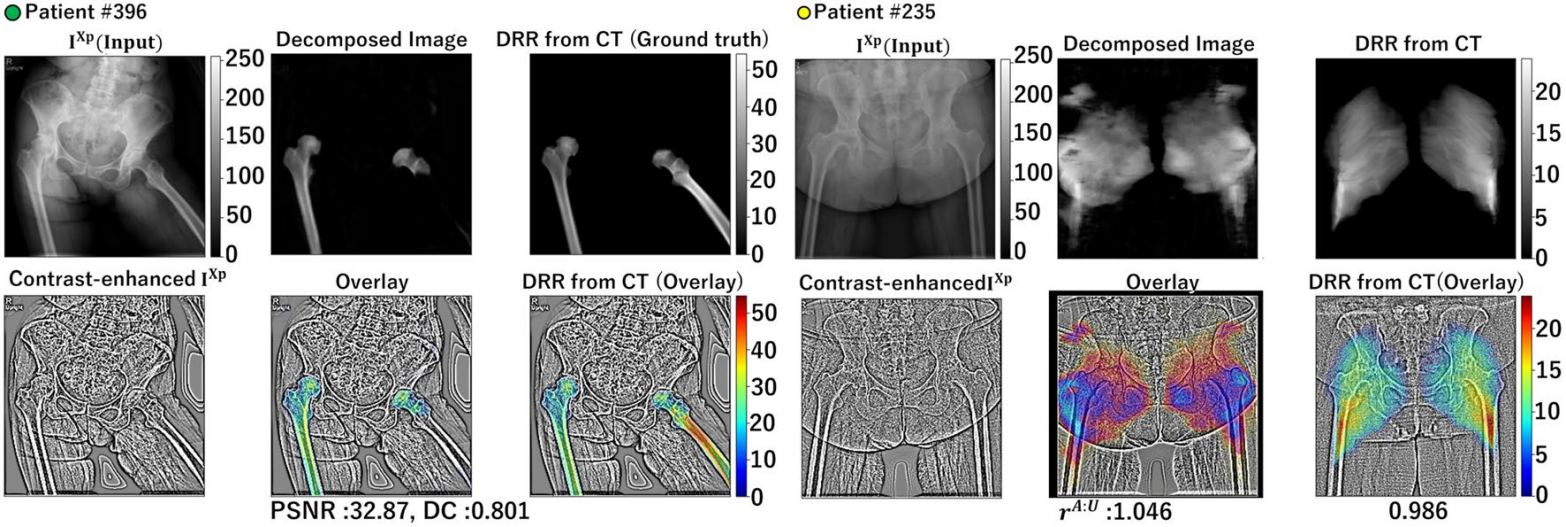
Representative failure cases decomposed from real X-ray image $$I^{Xp}$$. The first row shows $$I^{Xp}$$ with its contrast-enhanced images, the second row shows the decomposed images $${I}^{DRR}_n$$, and the third row shows the overlay of the decomposed images onto the contrast-enhanced images. $$r^{A:U}$$ denotes the ratio of the affected/unaffected AC-weighted volumes defined by Eq. (9).
